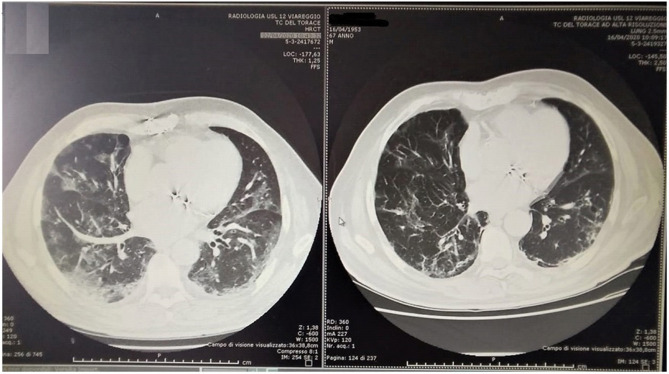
CT chest in 67 years old patient at T0 (Left) and at day 14 of treatment (Right).
